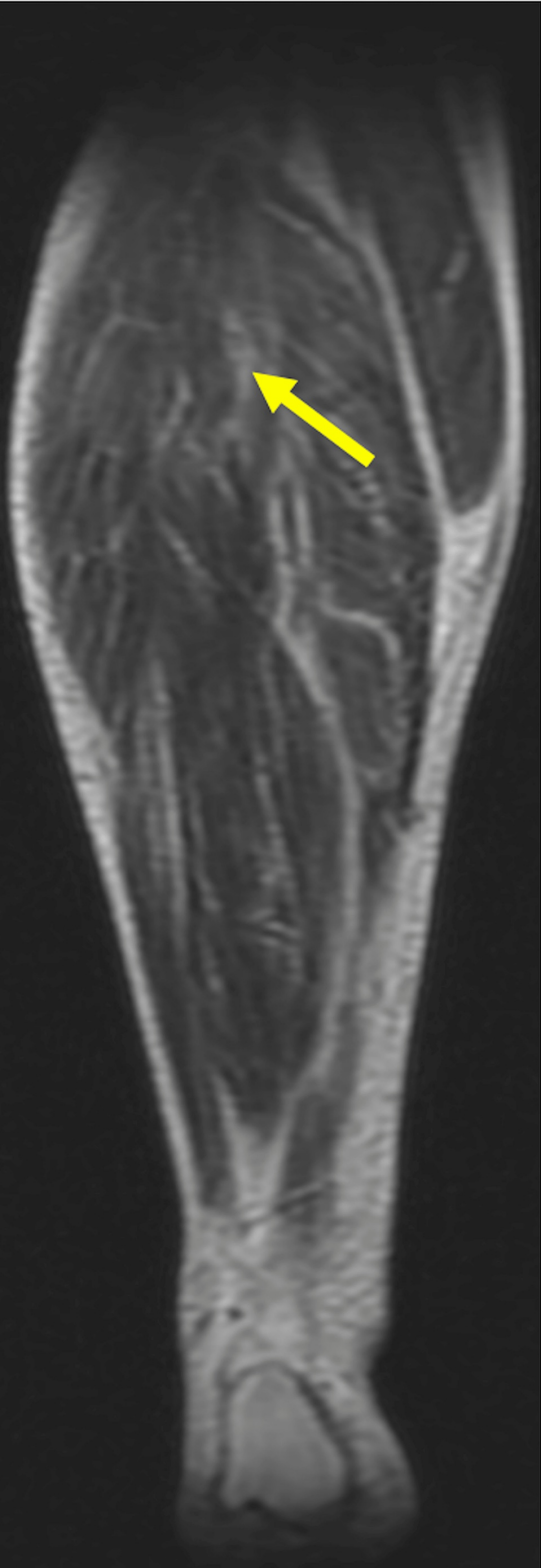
Coronal section T2-weighted sequence of the right lower limb. Coronal section T2-Weighted sequence of right lower limb showing extensive intramuscular hyperintensities in the anterior compartment (extensor digitorum longus), lateral (peroneus longus), and superficial posterior compartment (plantaris, gastrocnemius, and soleus) and intermuscular facial planes, predominantly in the proximal part. There are no e/o necrotic changes.
